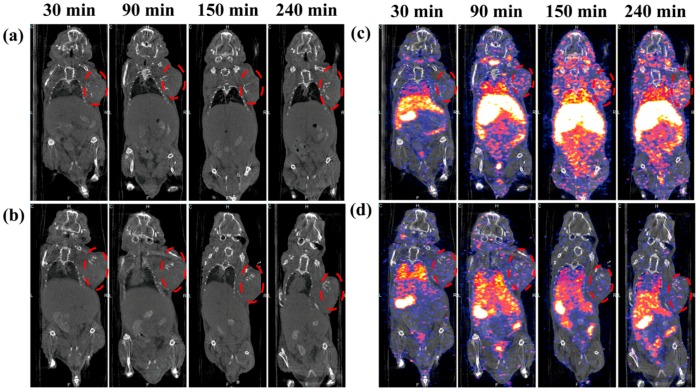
In vivo CT (a,b) and SPECT (c,d) images of tumors after intravenous injection of the {(Au°)_6_-G2-DTPA(^99m^Tc)-PEG-FA} (a,c) or {(Au°)_6_-G2-DTPA(^99m^Tc)-*m*PEG} (b,d) DENPs ([99mTc] = 740 MBq·mL^−^^1^, Au = 0.08 M, in 100 μL PBS) at different time points post-injection. The dashed red circles indicate the tumor sites (adapted from [73], ACS Applied Materials & Interfaces, 2016).
